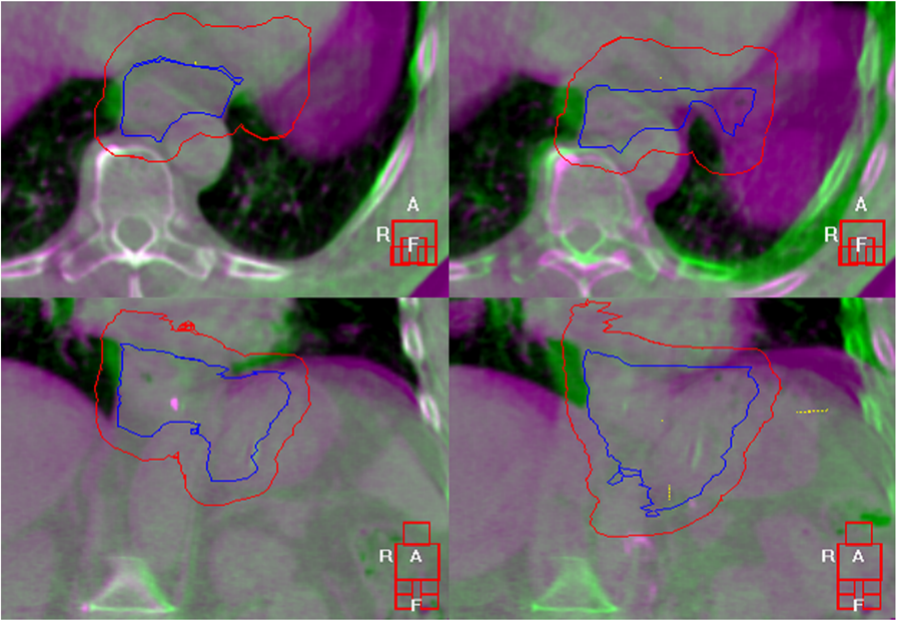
Example of inadequate target coverage after registration. Overlay of the pCT (purple) and CBCT (green) in XVI for patient 20, registered on the bony anatomy (left column) and the carina (right column). In axial (first row) and coronal view (second row). A = anterior, R = right, and F = inferior. Red contour = PTV, blue contour = CTV
